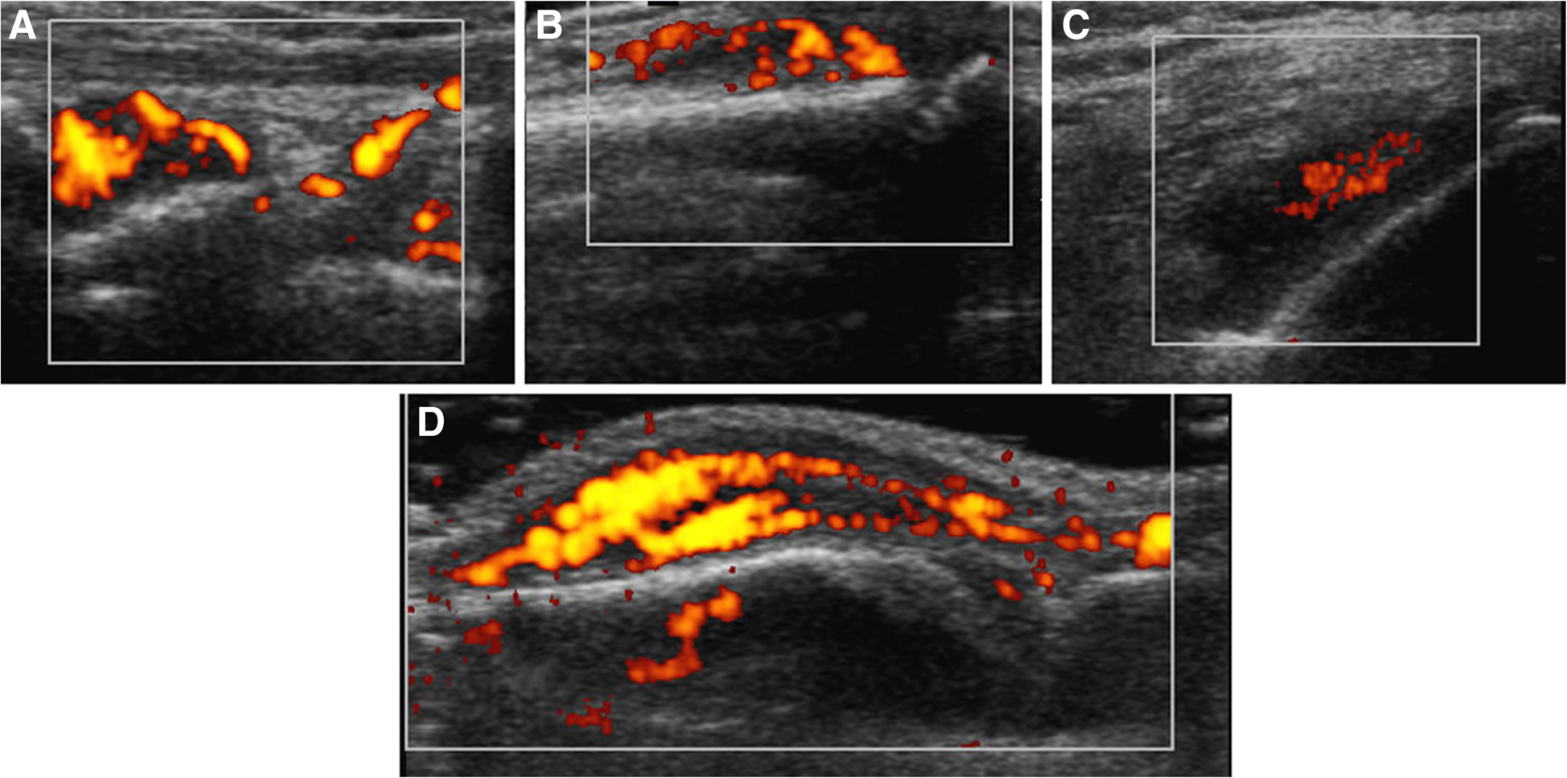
Representative images of ultrasound-defined synovitis in assessed joints. (A) Radiocarpal joint, longitudinal view: synovial hypertrophy (SH) grade 2 and power Doppler ultrasound (PDUS) signal grade 3. (B) Proximal interphalangeal joint, longitudinal view: SH grade 2 and PDUS signal grade 2. (C) Knee joint: parapatellar recess, transversal view: SH grade 2 and PDUS signal grade 2. (D) Metacarpophalangeal joint, longitudinal view: SH grade 2 and PDUS signal grade 3.
